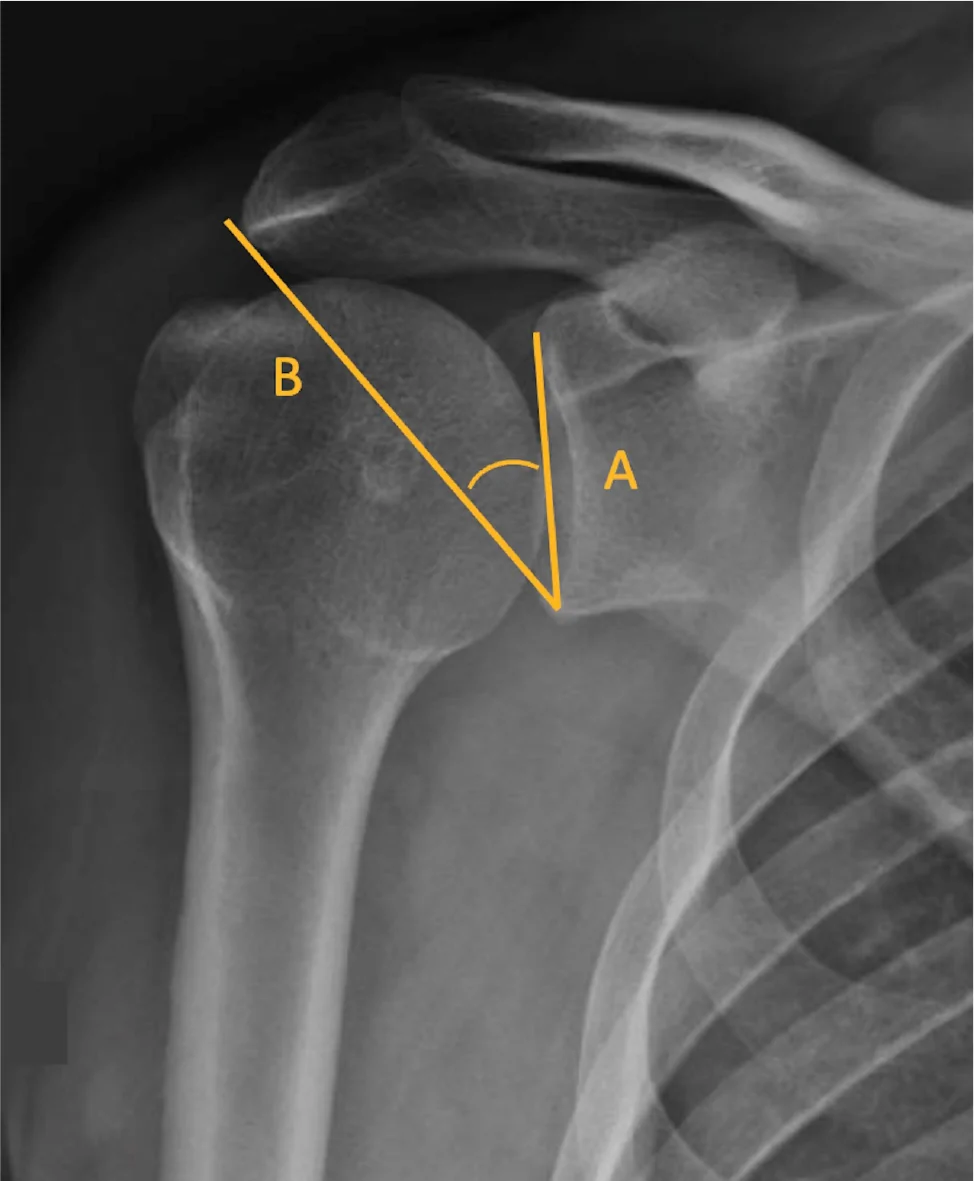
An AP X-ray view of the shoulder depicting the CSA. A: Line from
